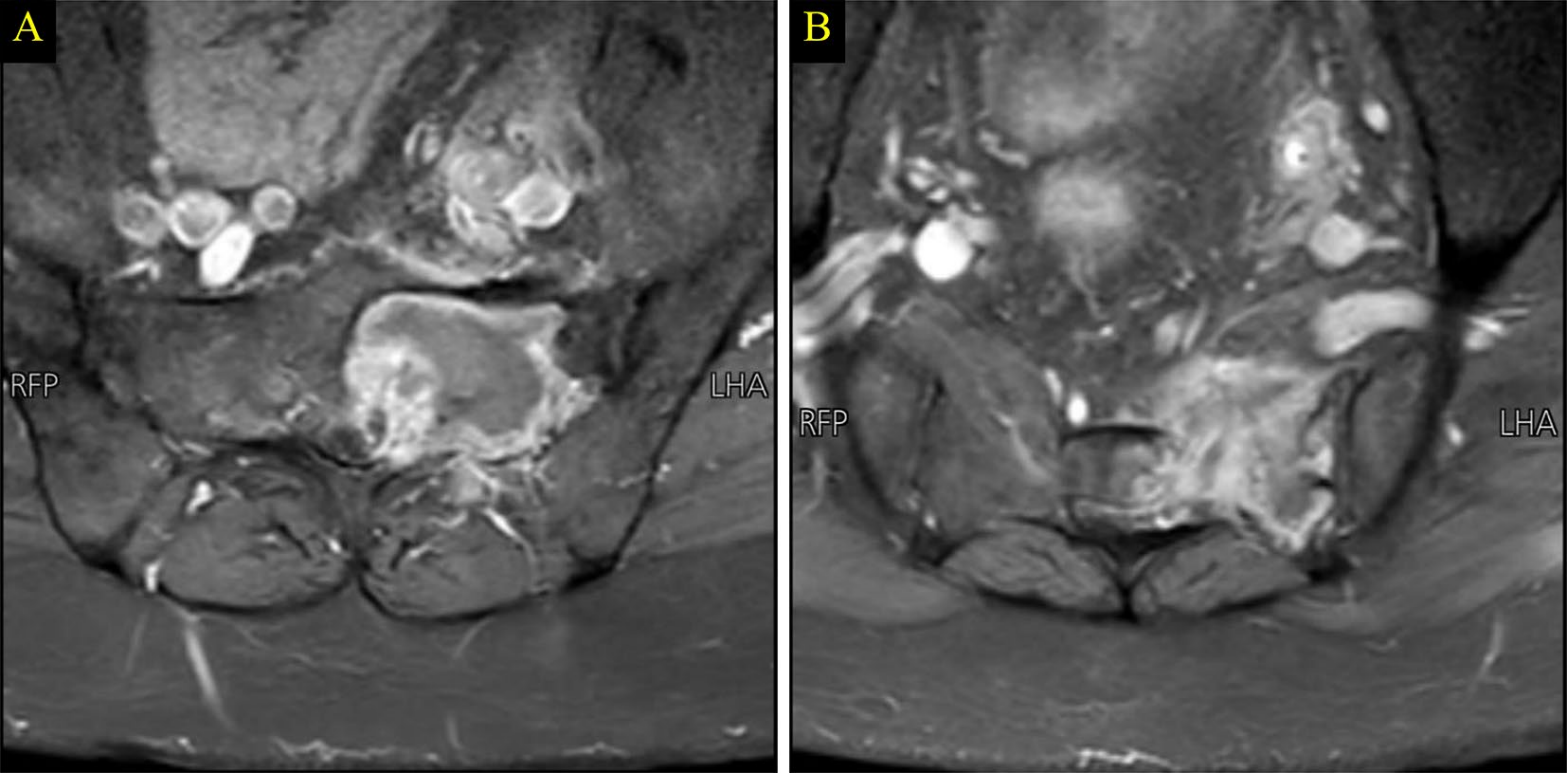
Multilevel axial T1 MRI post- contrast sequences reveal a large necrosis in the site of bony metastatic lesion indicating good response to the treatment
